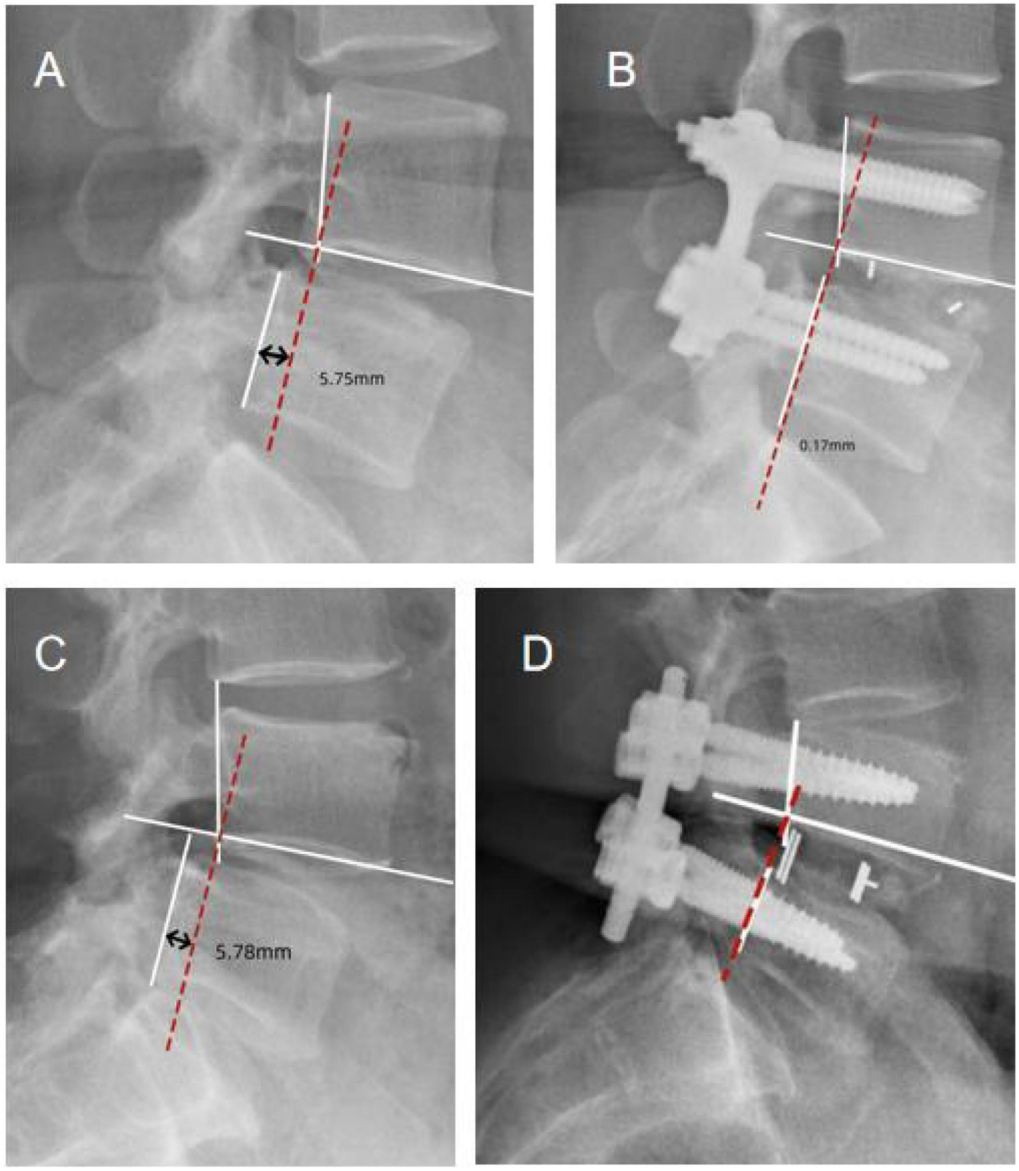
Translational motion measurement. The distance from the posterior vertebral line of L4 to the posterior edge of L5 on the upper endplate of L5 was measured in flexion and extension radiographs. [(A) Unilateral laminotomy, Preoperative; (B) unilateral laminotomy, Postoperative; (C) conventional laminectomy, Preoperative; (D) conventional laminectomy, Postoperative].
